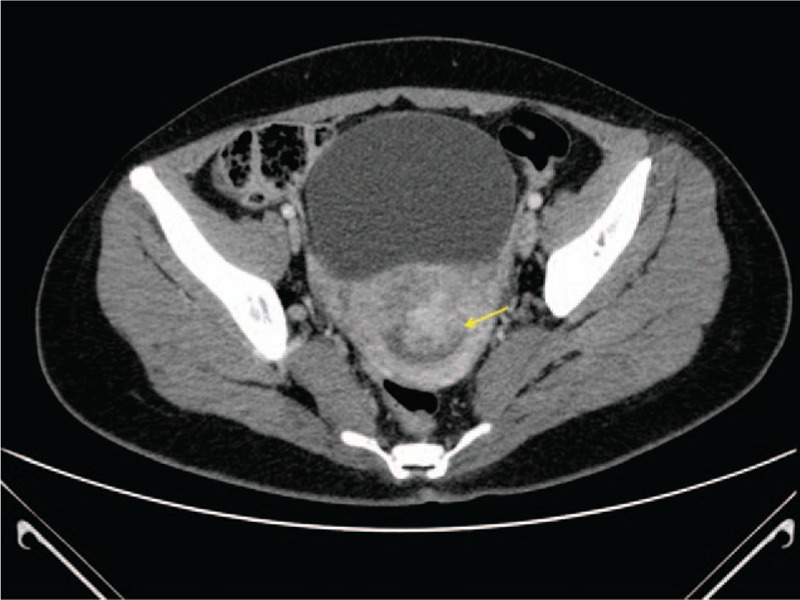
Computed tomography scan showing an intrauterine mass (yellow arrow).
